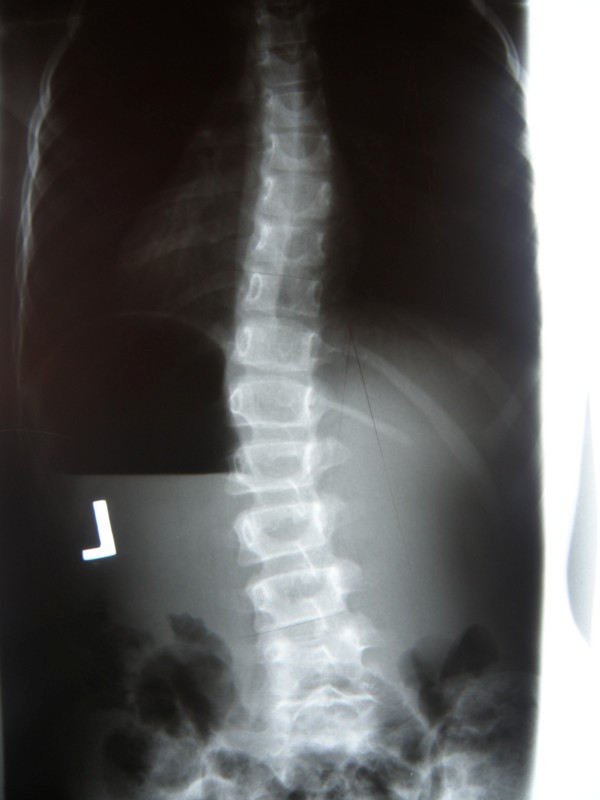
Radiography after treatment.
